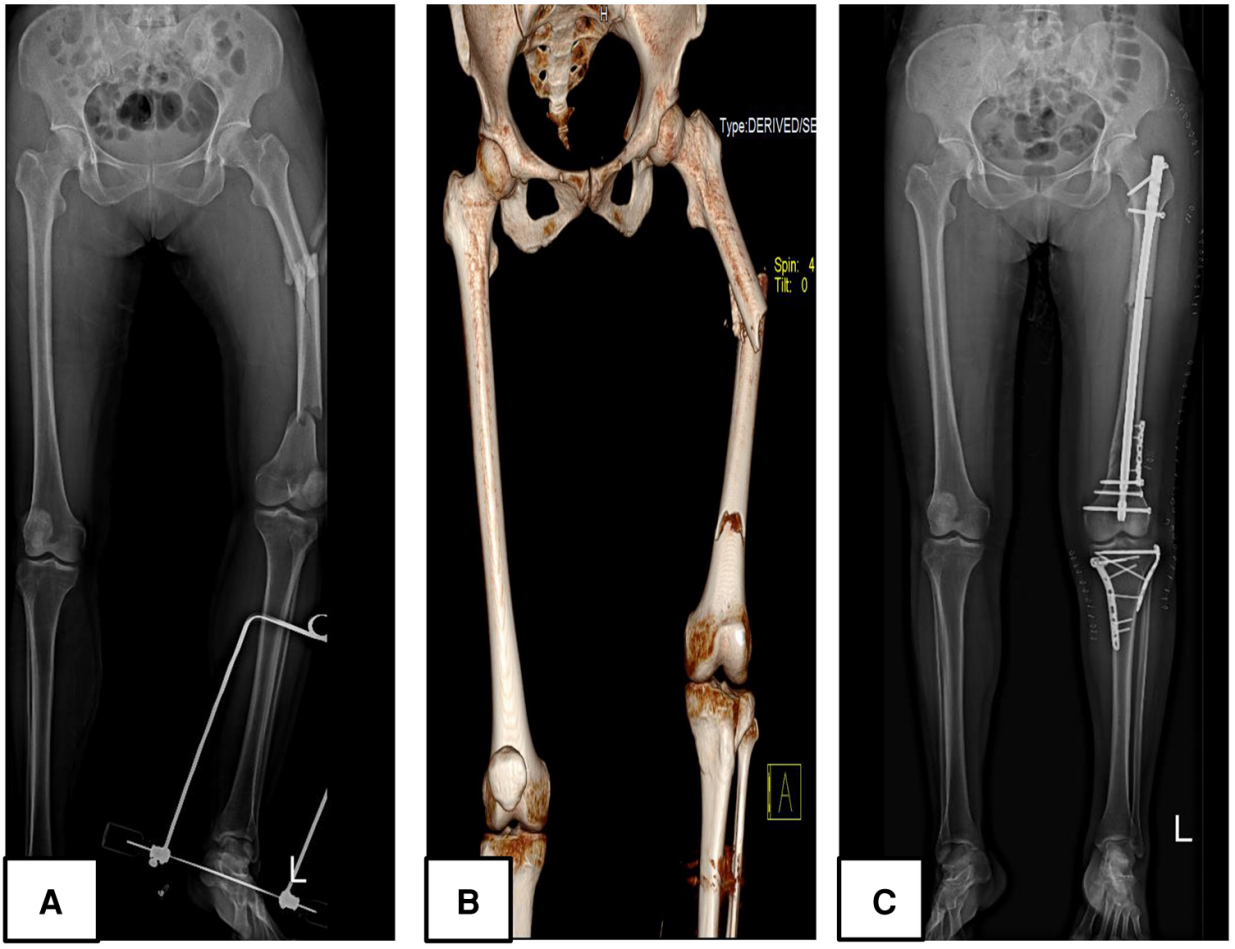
A 48 years-old female patient (patient number 32) who sustained a Fraser type IIA floating knee following a road traffic accident managed surgically; (A,B) represent preoperative plain radiograph and 3D reconstruction CT images respectively; (C) displays a postoperative plain radiograph of the lower limb, the femur was managed with an intramedullary nail combined with a reconstruction plate and the tibia was treated with a dual plate.
